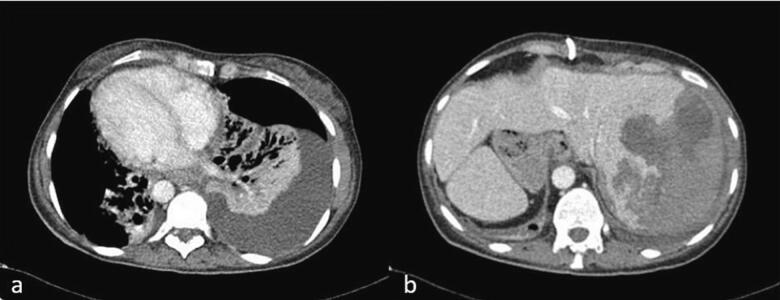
CT scan showing hospital course complications. (a) Left-sided pneumonia, (b) large subcapsular hematoma in the liver from PTC.
